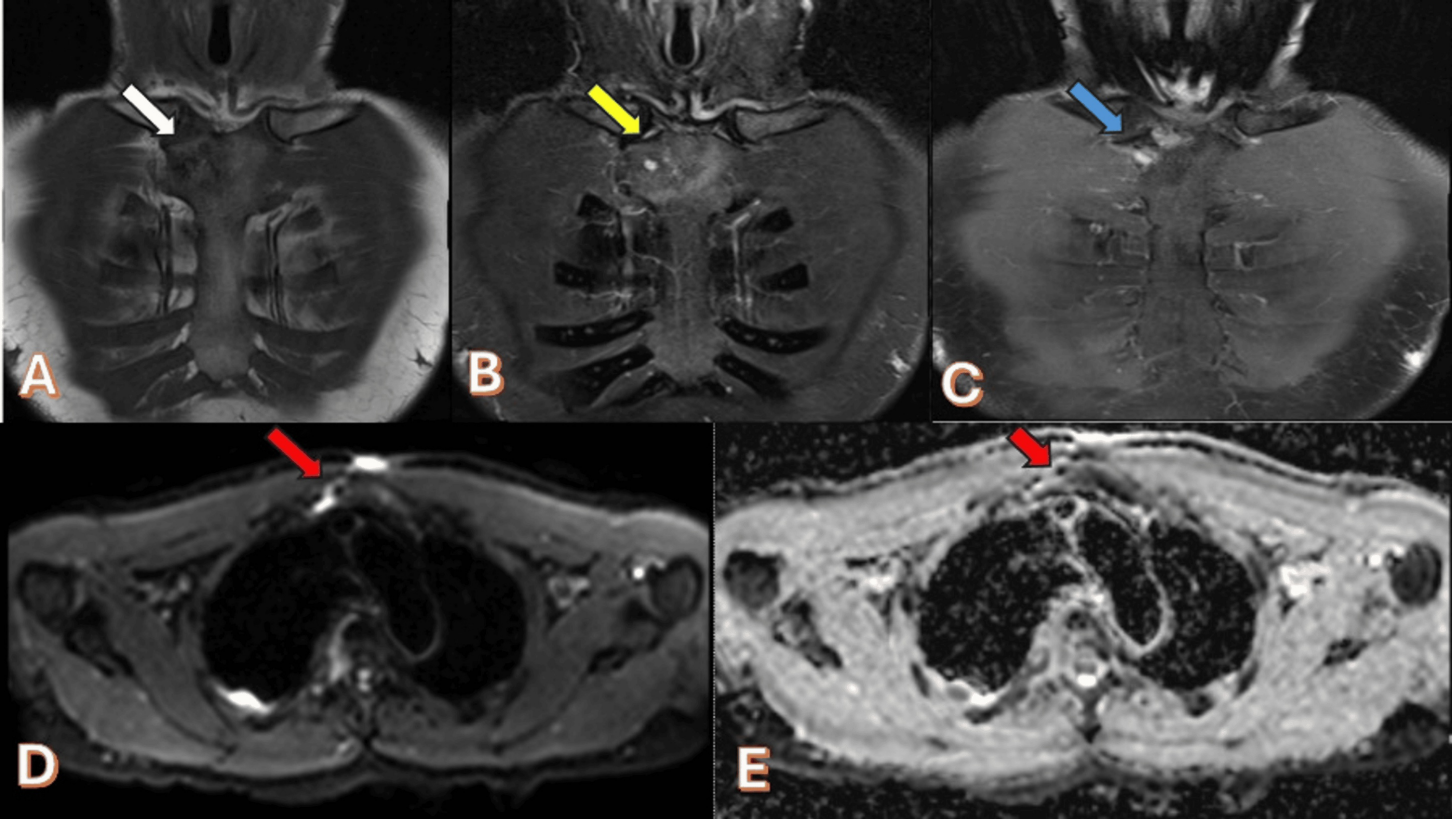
(A) Coronal precontract T1WI shows the following: Ill-defined iso- to hypointense lesion seen in the right lateral aspect of the manubrium (white arrow) demonstrating heterogenous predominately high signal intensity seen in coronal T2WI (yellow arrow) (B). The lesion shows post-contrast enhancement noted in T1 coronal fat sat post-contrast image (blue arrow) (C). There is an area of diffusion restriction noted as high signal in DWI and low signal in ADC in (D) and (E), respectively (red arrow) T1WI: T1-weighted Image; T2WI: T2-weighted Image; DWI: diffusion-weighted image; ADC: apparent diffusion coefficient
